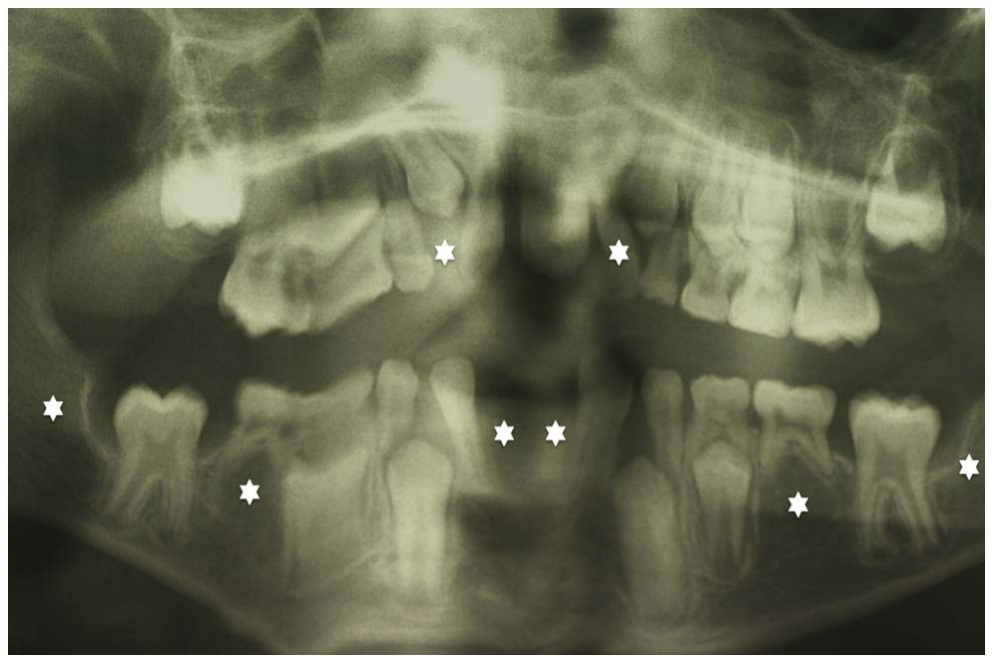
Dental anomalies encountered in Coffin-Lowry syndrome. This panoramic radiograph shows oligodontia (more than six permanent missing teeth (white stars: 12, 22, 41, 31, 45, 35, 47, 37). The deformations observed on this panoramic radiograph are linked to movements during the X-Ray acquisition process and difficulties for handicapped patient to remain still during the procedure.
